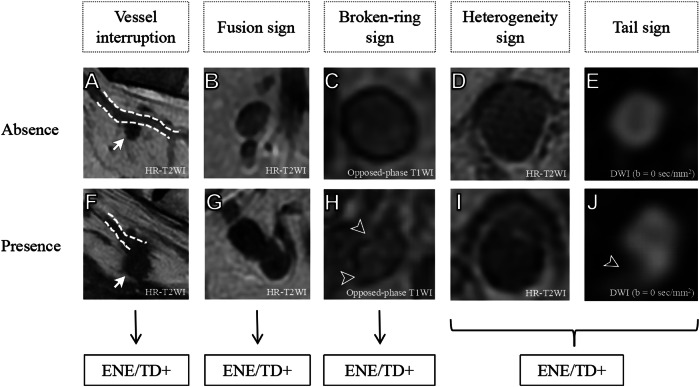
Representative images indicating the absence (A–E) or presence (F–J) of extranodal extension or tumor deposits (ENE/TD). A, F Vessel interruption by nodes: A absent, F present; dashed lines indicate vessels, arrows indicate nodes. B, G Fusion of nodes: B absent; G present. C, H Broken-ring sign: C absent; H present; arrowheads in H indicate broken capsule of the node. D, I Intra-nodal heterogeneity: D absent; I present. E, J Tail sign: E absent; J present; arrowhead indicates tail-like high signal attached to the node. DWI, diffusion-weighted image; HR-T2WI, high-resolution T2-weighted image; T1WI, T1-weighted image. Used with permission of Radiological Society of North America, from [27]; permission conveyed through Copyright Clearance Center, Inc
